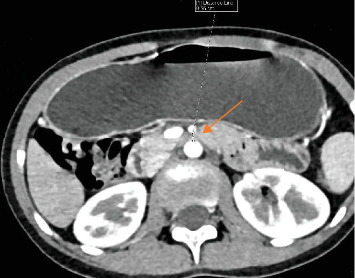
Abdominal CT scan showing a short AMD (5.6 mm).
